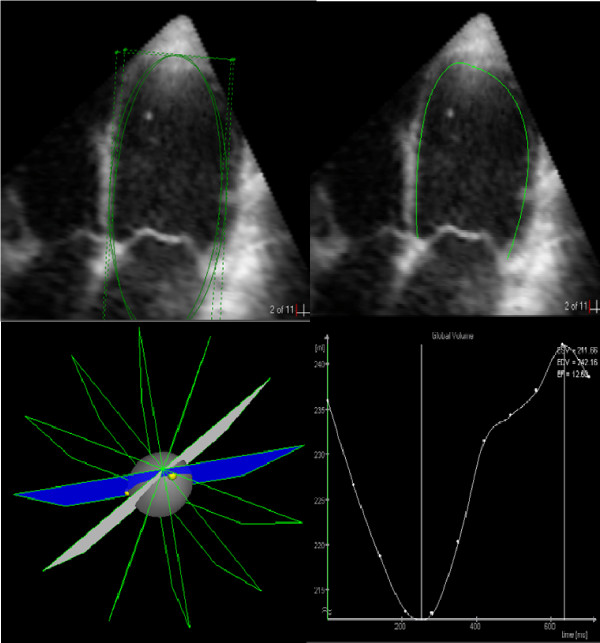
Analysis of LV volumes using 3D-echocardiography. In the selected LV image (upper left), the algorithm (4D LV-Analysis CRT 1.0, TomTec) provides semi-automated delineation of the endocardial border (upper right). By superposition of LV contours from 6 insonation planes (lower left) in 3D space, the LV volumes are calculated and the graphic presentation of LV volume changes in time can be obtained (lower right).
